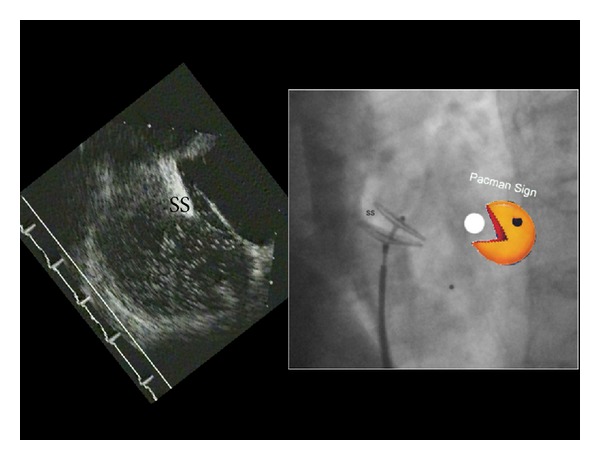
Correct placement of an Amplatzer PFO occluder ascertained by the Pacman sign. The transesophageal echocardiogram at the left is depicted in the projection usually used on fluoroscopy during implantation. The septum secundum (SS) has to be bitten into by the left more cranial parts of the occluder disks. This reminds us of Pacman biting into a dot.
